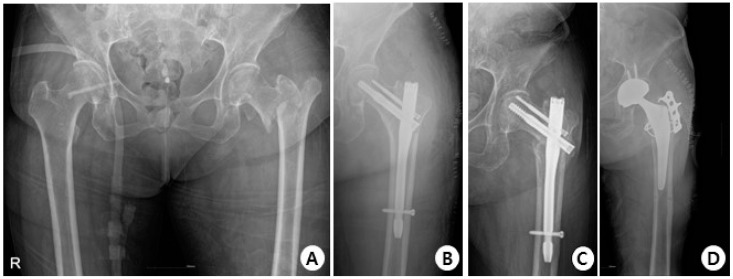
(A) Total hip AP X-ray of a 77-year-old female diagnosed with an intertrochanteric fracture of the left hip. (B) Immediately post-operation, reduction was performed using the Affixus hip fracture nail, but there appeared to be a mismatch in rotation. (C) The postoperative six-month X-ray shows reduction loss and the progression of varus deformity, with the lag screw experiencing cut-out. (D) X-rays show the revision surgery performed with arthroplasty.
